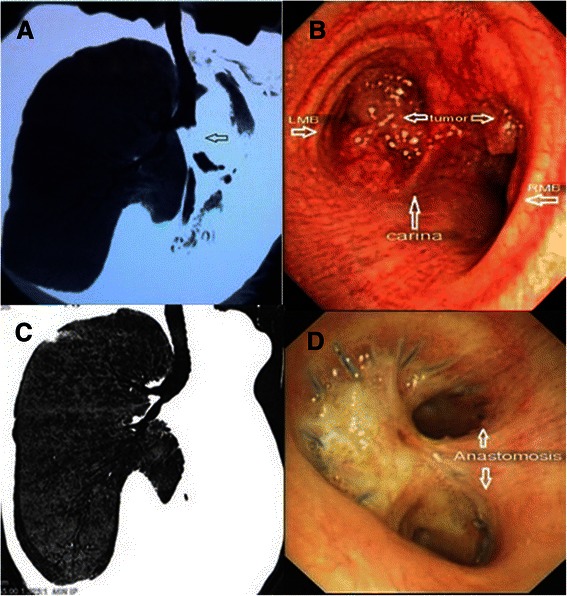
a The tumor in the left main bronchus extended up to the bifurcation of the upper and lower lobe orifices. Atelectasis of the left lung was seen. b Tumor involved the carina and proximal part of the right main bronchus. c CT showed an unobstructed airway at 19 months after the operation. d Bronchoscopy reveald an intact anastomosis, smooth mucosa
